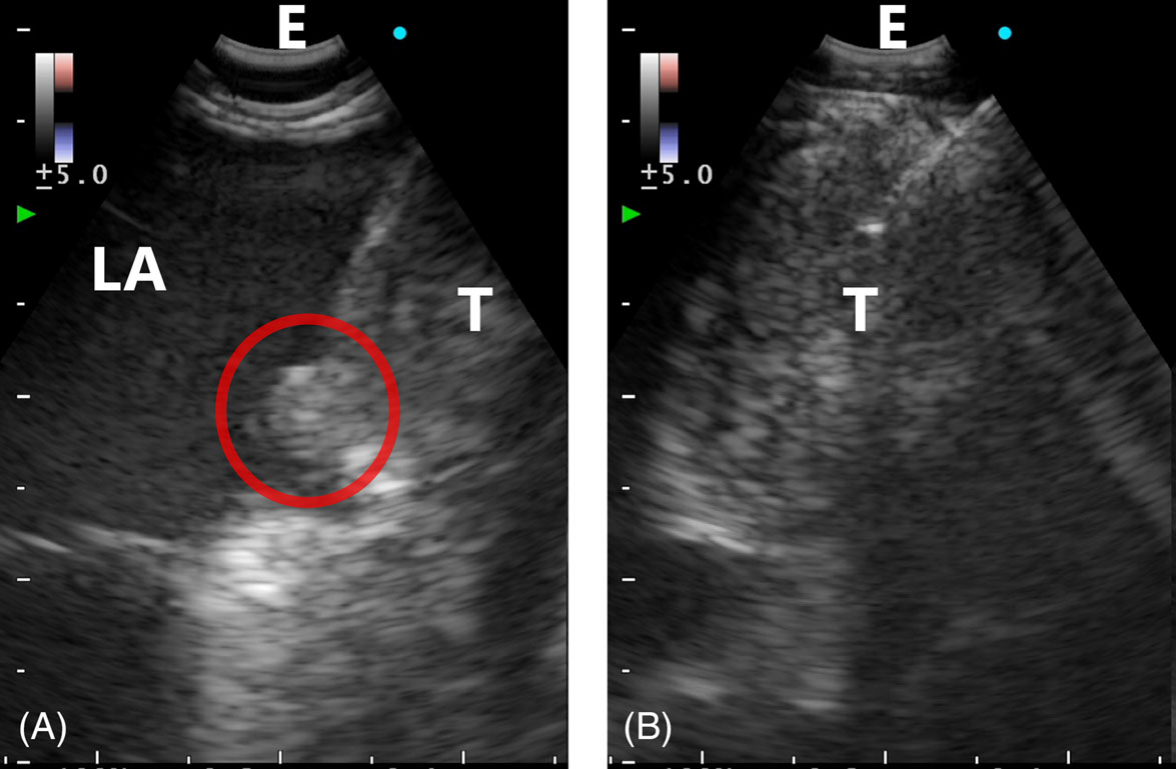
EUS‐B‐FNA findings. EUS‐B‐FNA findings show tumour invasion into the left atrium (A) and fine‐needle aspiration (B) of the mediastinal tumour adjacent to the heart. The red circle indicates tumour invasion. EUS‐B‐FNA, transesophageal ultrasound‐guided bronchoscopic aspiration
